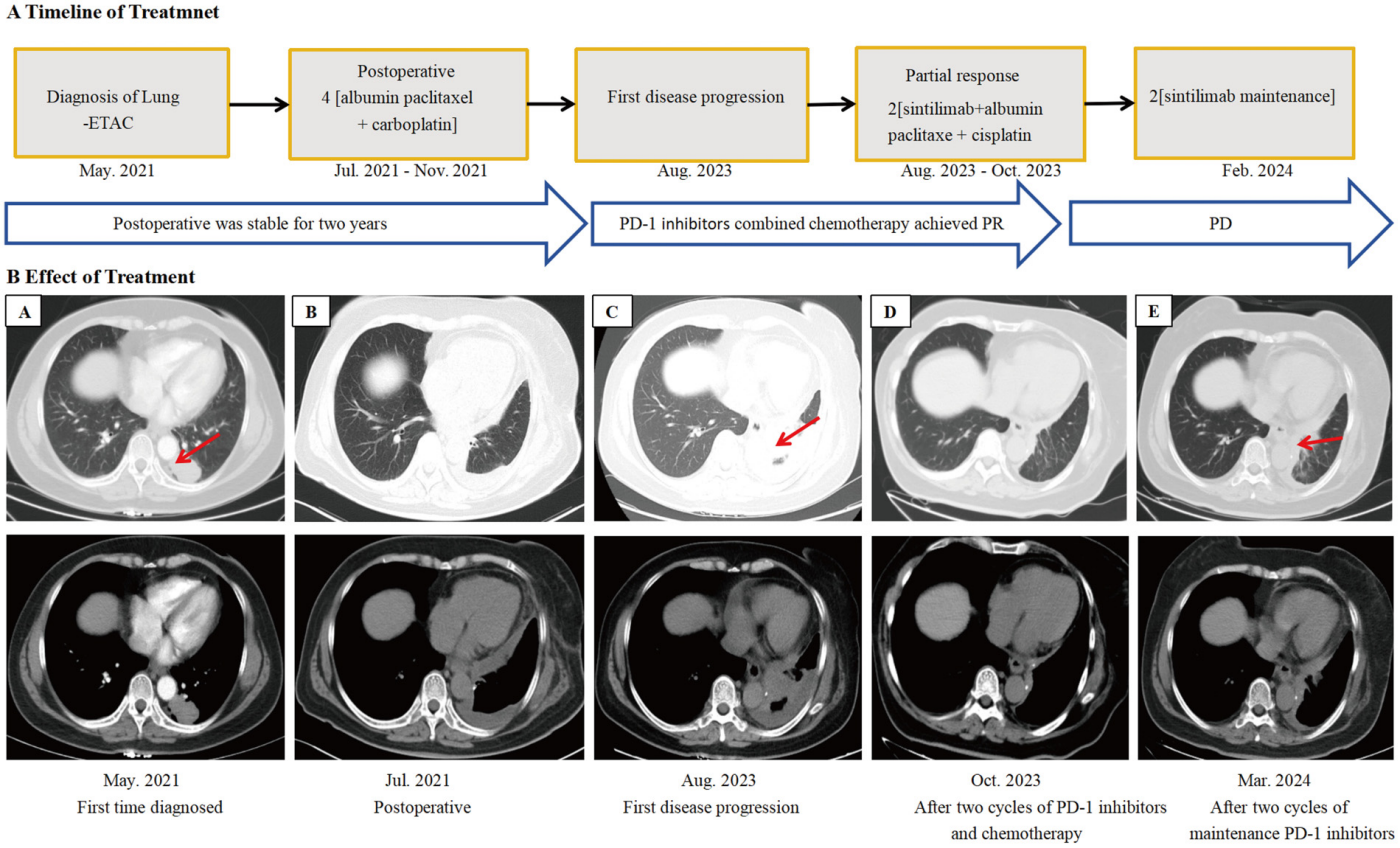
Imaging changes during treatment. CT images demonstrating diagnosis, treatment responses, and progression. (A) Lung-ETAC was first diagnosed (May 2021), enhanced CT scan showed a 2.6x3.8cm pulmonary nodule of the left lung. (B) Postoperative (Jul 2021). (C) Progression after postoperative, chest CT scan showed high-density shadows of bronchia in the left lung and larger than before (Aug 2023). (D) Partial remission was achieved after two courses of PD-1 inhibitors in combination with chemotherapy (Oct 2023). (E) Progression after two courses of maintenance immunotherapy (Mar 2024). PR, partial response; PD, disease progression.
